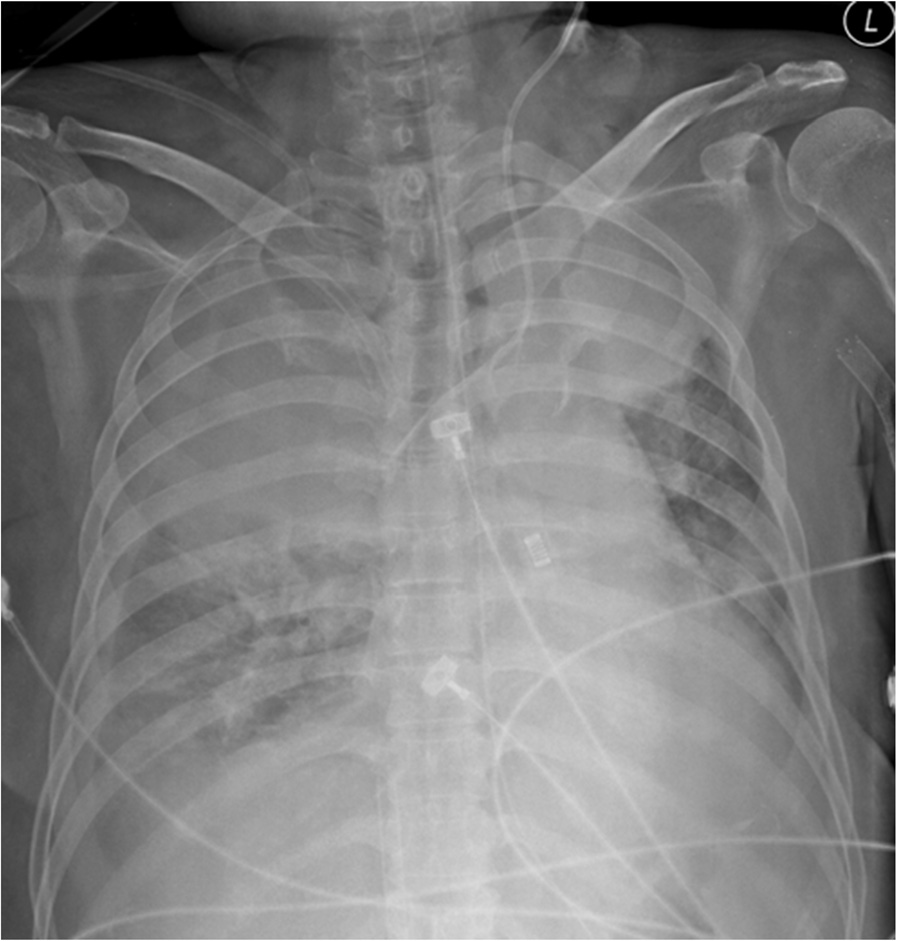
The chest x-ray after instituting ECMO shows diffuse infiltration in both lungs that developed after massive hemoptysis.
